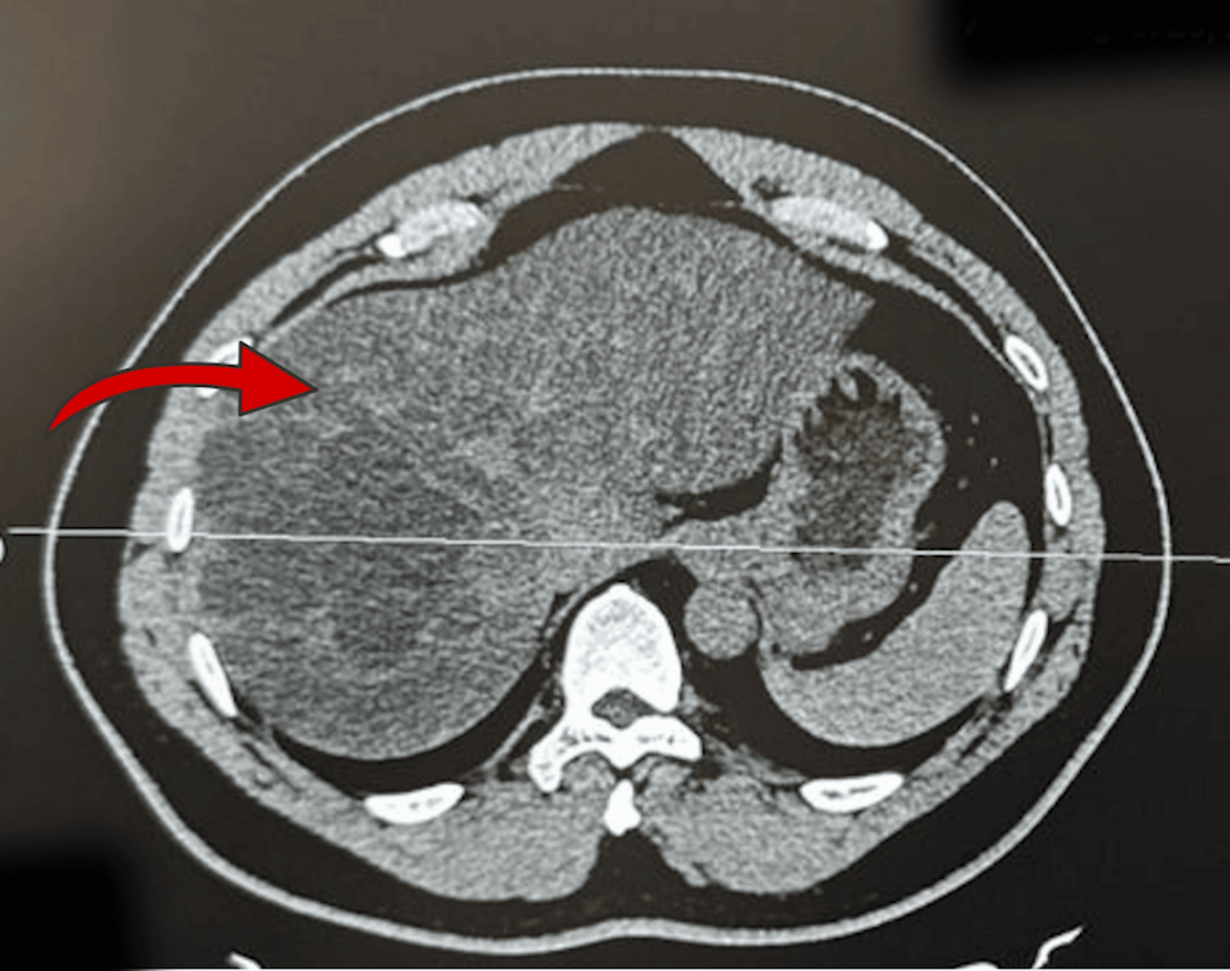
Computed tomography (CT) of the abdomen (axial view) Axial CT image showing the cystic lesion involving segments VII and VIII of the liver, without enhancing solid components.
